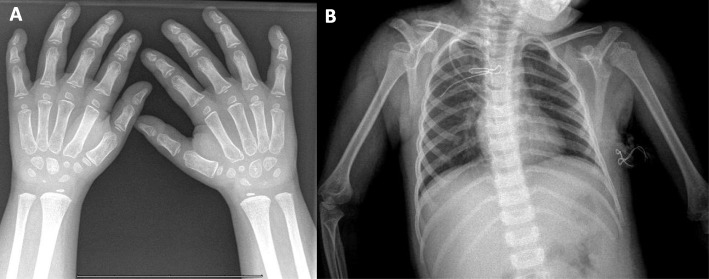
Characteristic signs of spondylo-epiphyseal dysplasia on chest-humeri and arm X-rays. Hand (a) and Chest-humeri (b) X-rays of *Patient 1* are presented. Short and broad metacarpi and phalanges, porotic bones, hypoplastic bony islands of the basal phalanges below the epiphyseal plates are clearly visible. The epiphyses of the humeri are broadened, the vertebrae have normal morphology
